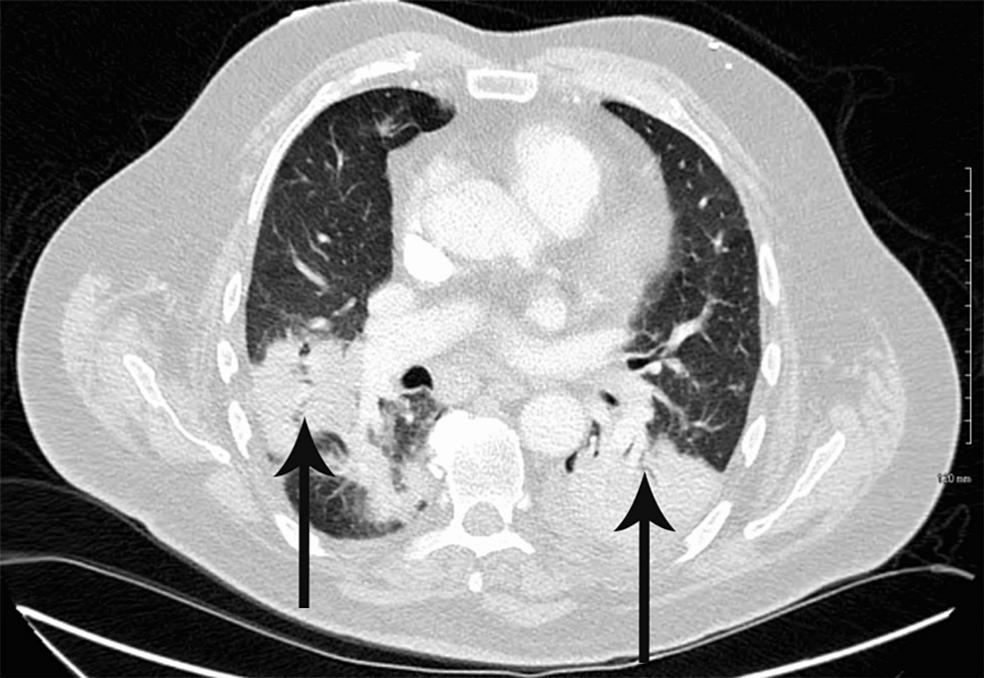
CT scan of the chest with contrast showing bilateral lung opacities (arrows) CT: computed tomography
